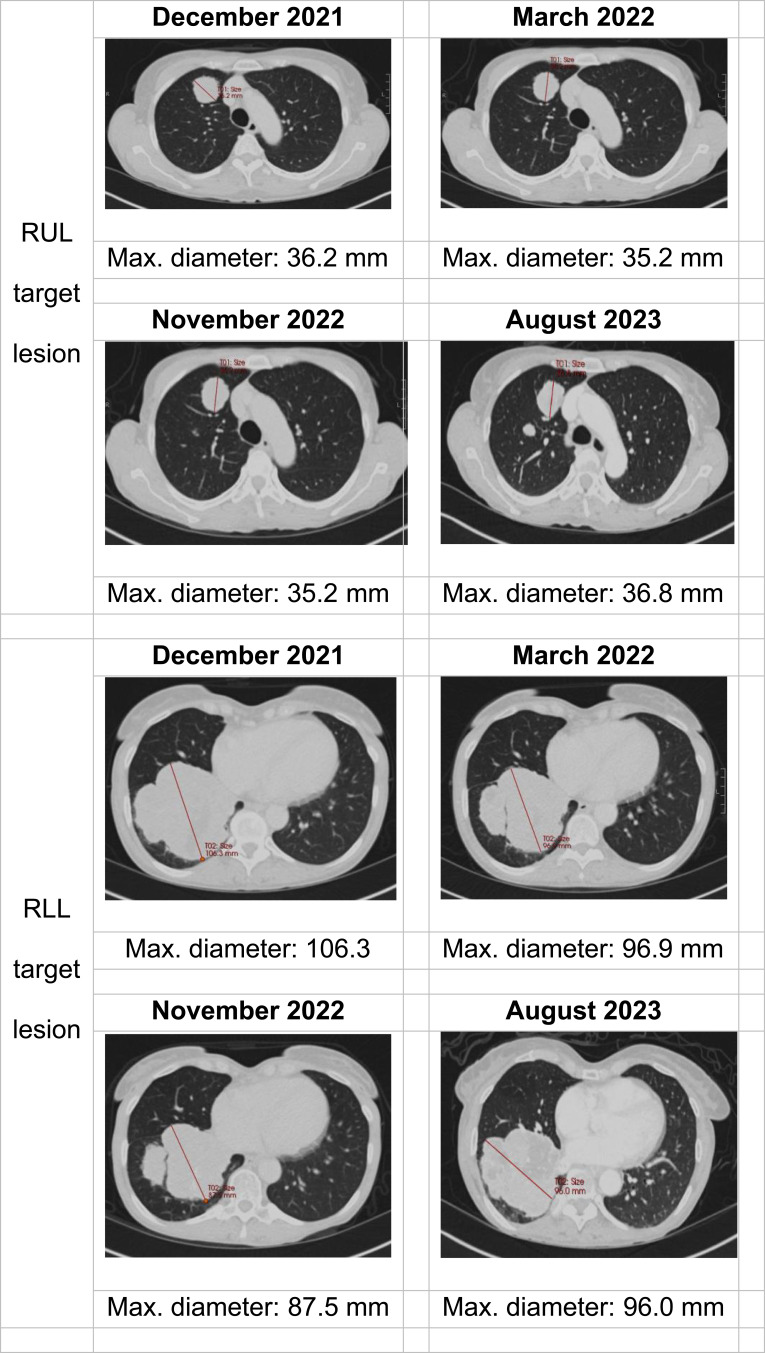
Serial computed tomography imaging of the chest. The images portray the target right upper lobe (RUL) and right lower lobe (RLL) lesions at baseline (progression on previous ATP-competitive FGFR inhibitor, prior to starting futibatinib) at the first response evaluation time point (3 months after commencing futibatinib), at the time of maximum tumour shrinkage (12 months after commencing futibatinib), and at the present time (20 months after commencing futibatinib).
